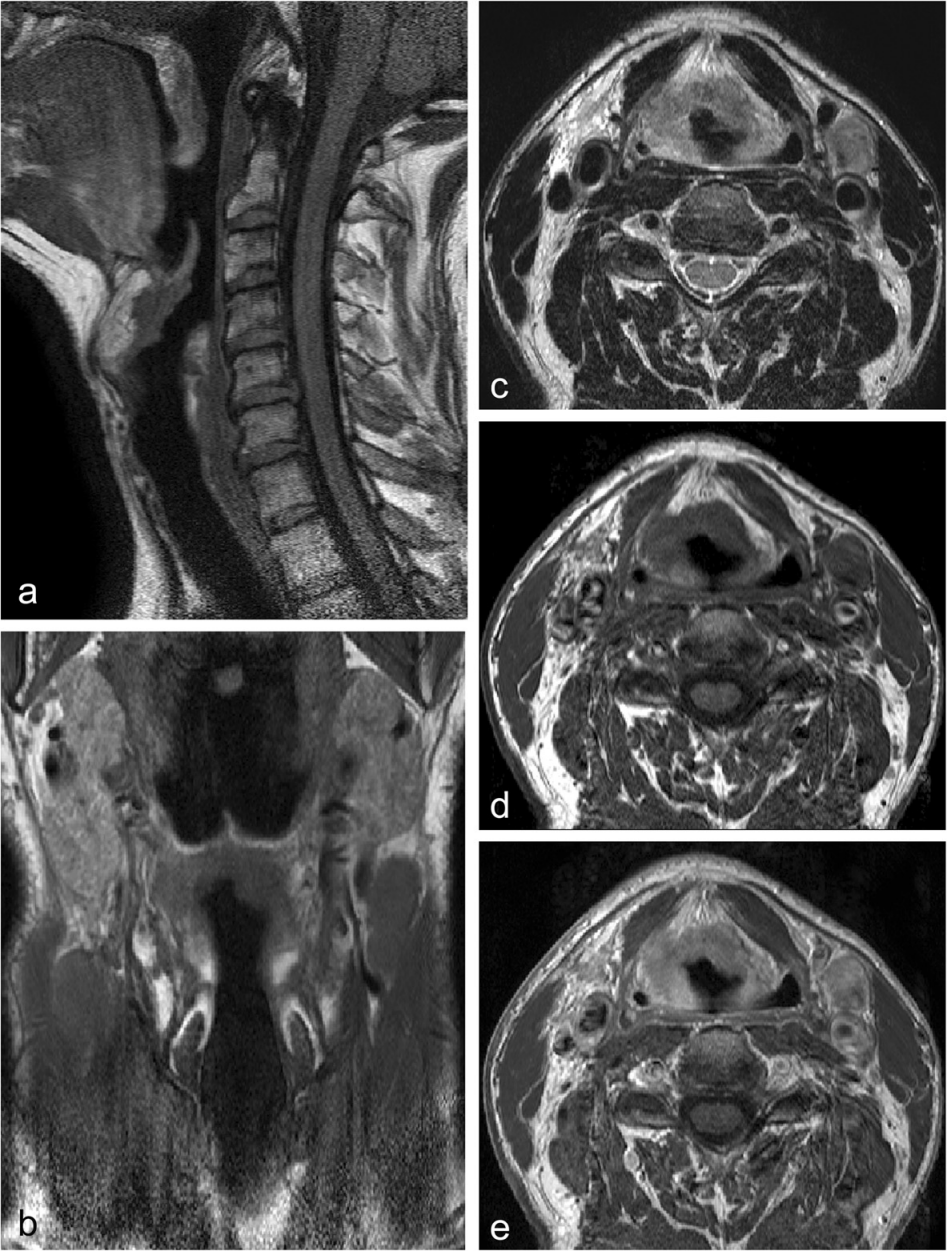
MR-images of the larynx acquired in a radiotherapy mask. a:T1-weighted sagittal view, b: T1-weighted coronal view. Transversal views: c: T2-weighted, d: T1-weighted, e: T1-weighted + gadolinium.
